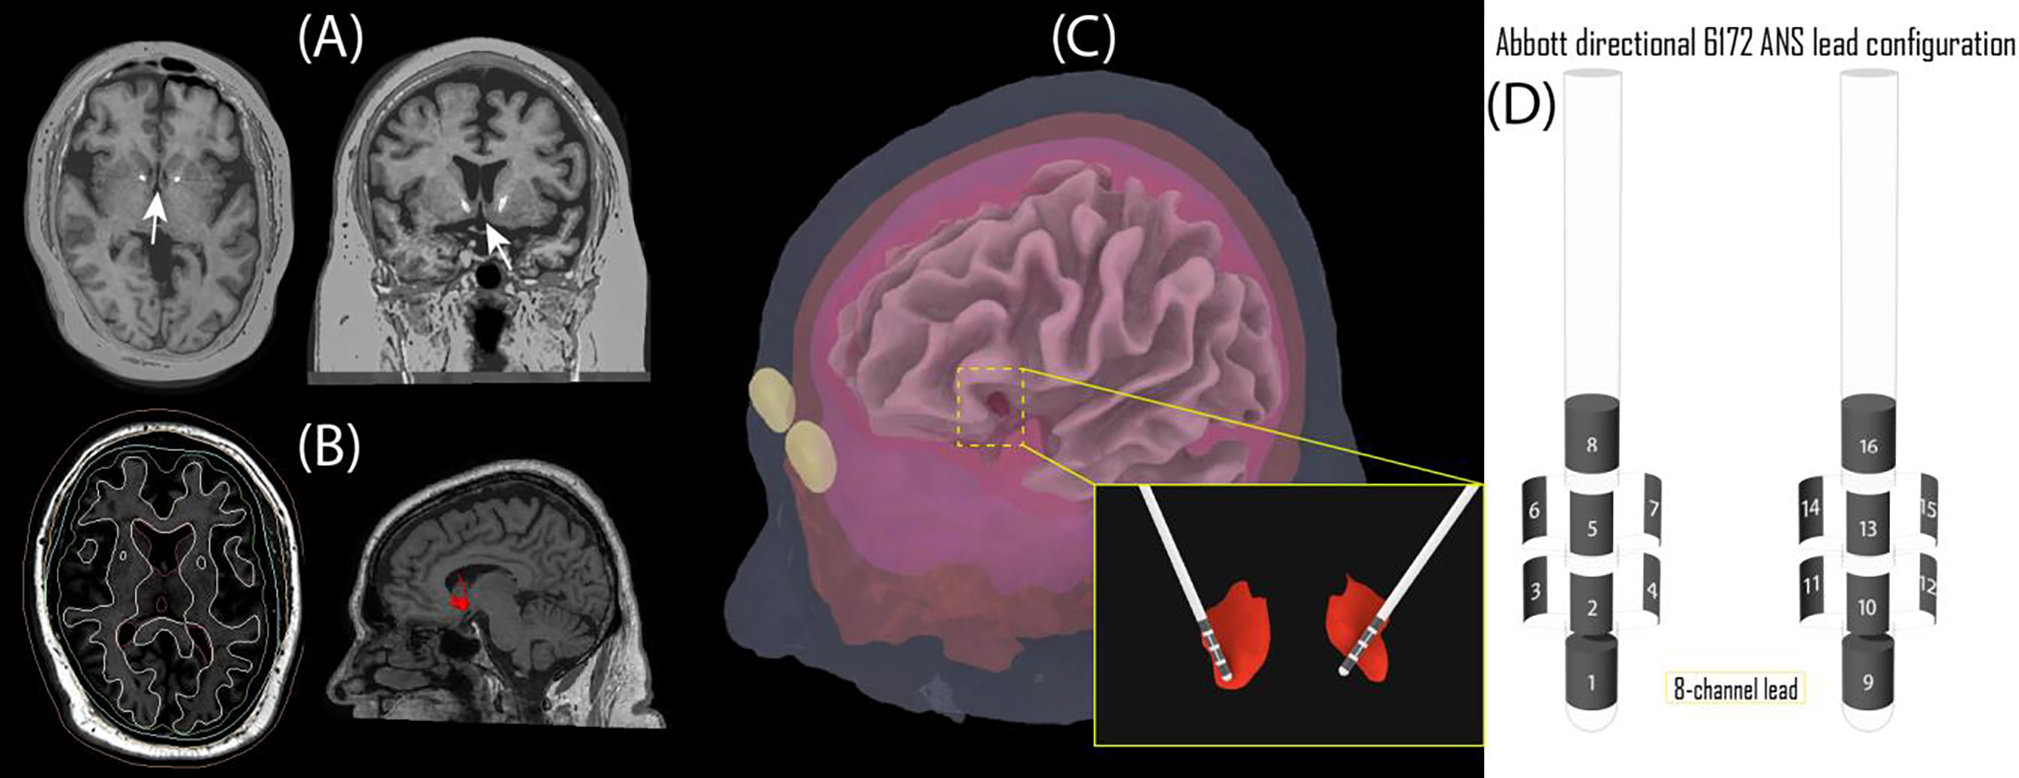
Steps of image segmentation and model creation. (A) post-op CT images were fused with pre-op MRI for electrode localization. (B) MRI images were segmented in SimNIBS to create masks of gray matter, white matter, CSF, skull, scalp, and ventricles. NAcc was segmented using Freesurfer and FSL. (C) 3D surfaces of different head compartments were created from smoothed masks. DBS leads were created around the centerline of lead artifact extracted from CT images. (D) Abbott directional 6172 ANS lead configuration.
